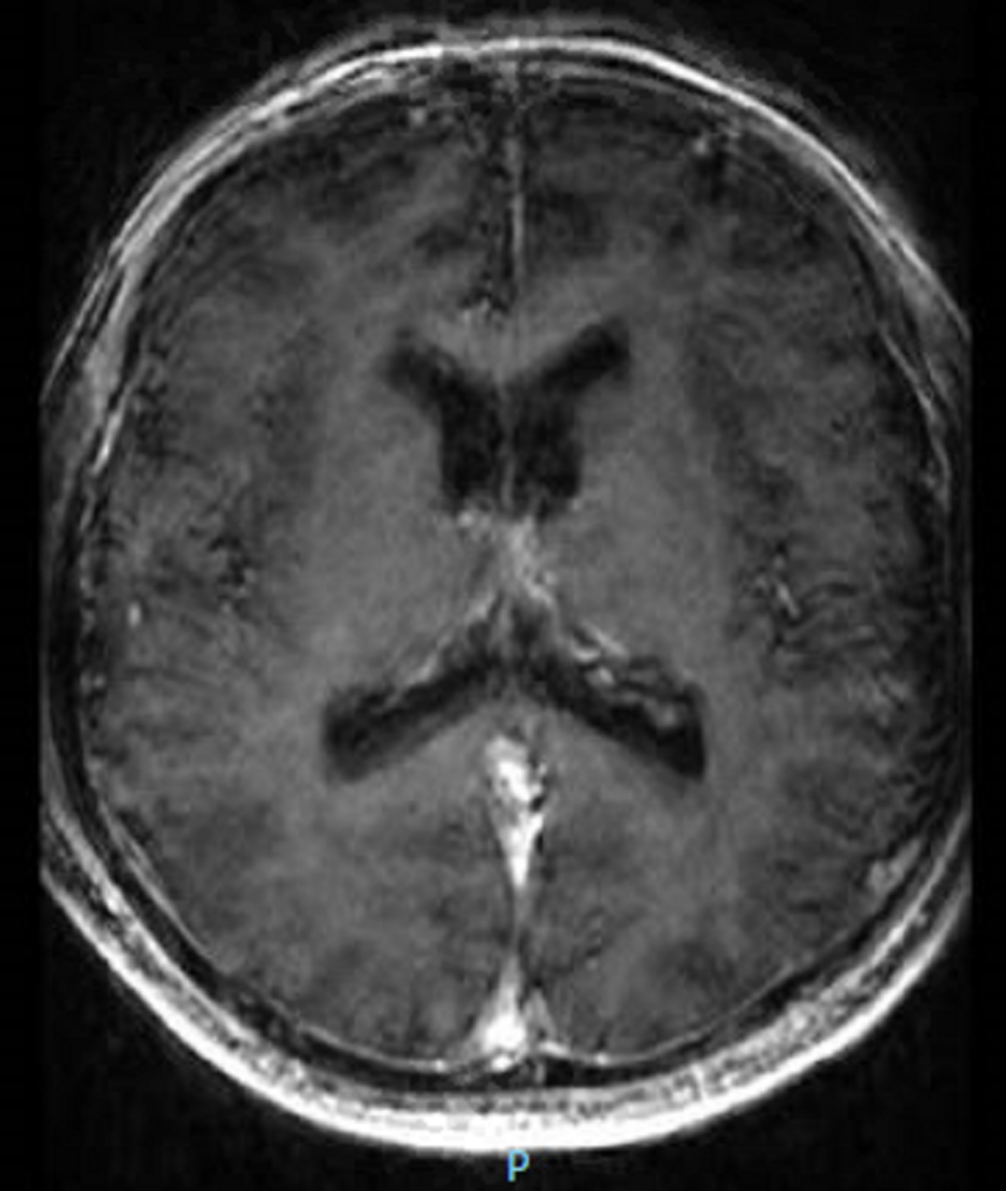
Computed tomography scan of the head
